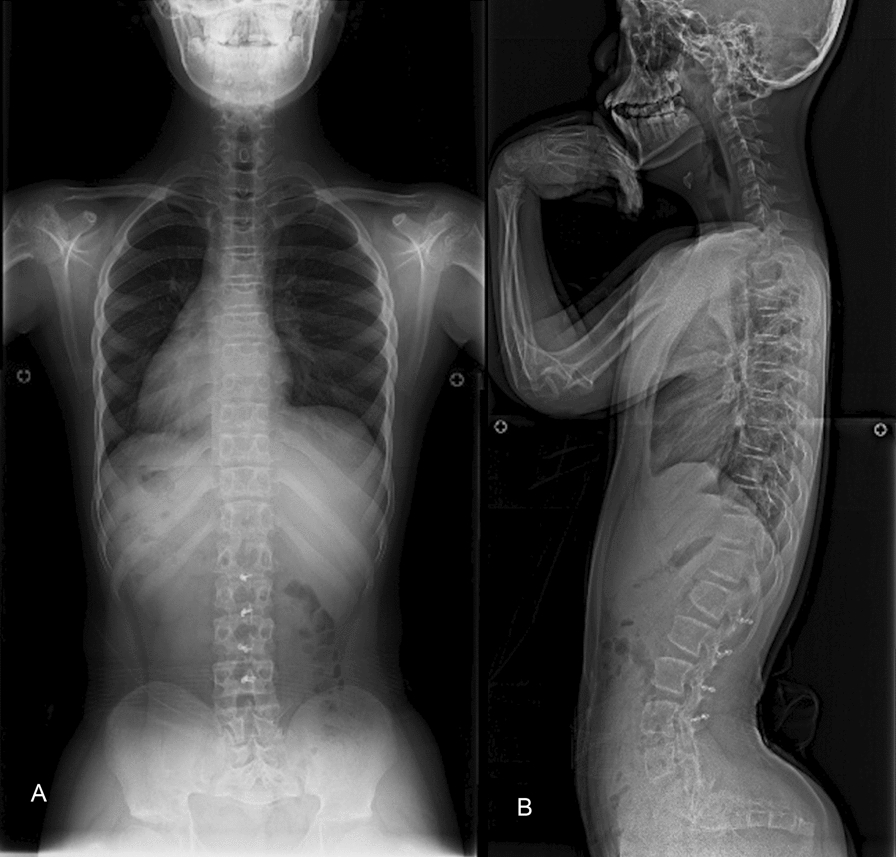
Postoperative whole-spine X-ray images (A: lateral view, B: frontal view). No remarkable kyphotic deformity or alignment changes were observed
